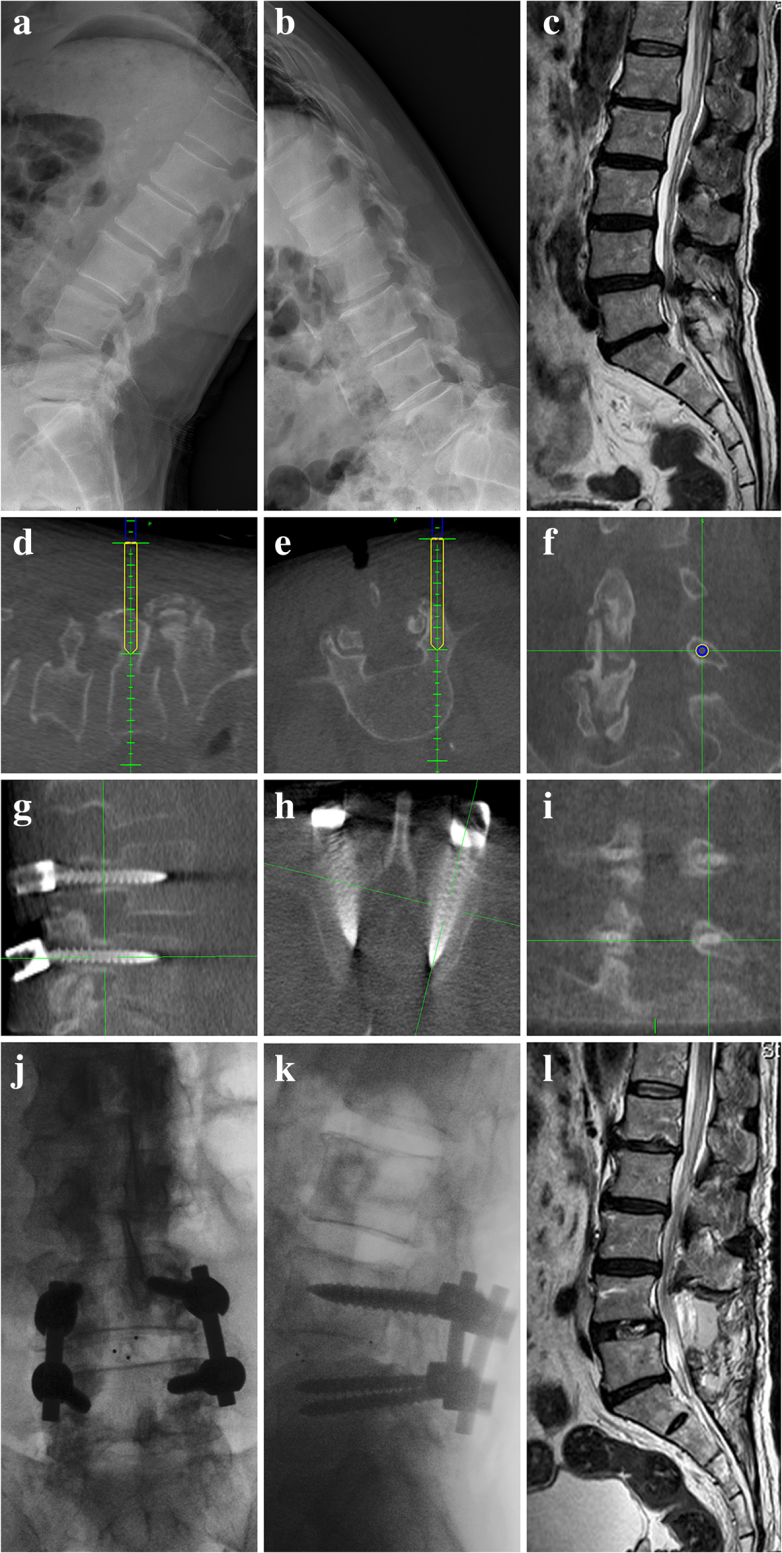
A 59-year-old woman presented with lumbar and lower extremities pain for 3 years. a–c Preoperative spinal radiography and magnetic resonance imaging (MRI) showed lumbar spondylolisthesis and disc herniation. d–f Images of the navigation screen. g–i Images of the O-arm screen obtained from the second intraoperative O-arm scan. j, k Images of the O-arm screen obtained from the third intraoperative O-arm scan. l Postoperative MRI
